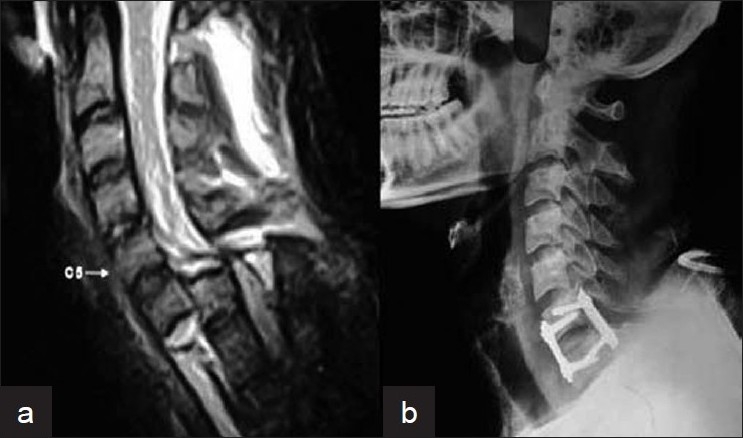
(a) Pre-op sagittal MRI T2W image of C6-C7 dislocation in whom reduction could not be achieved despite on-table and post-operative traction. (b) Post-operative X-ray of cervical spine (lateral view) showing posterior facetectomy and global fixation
